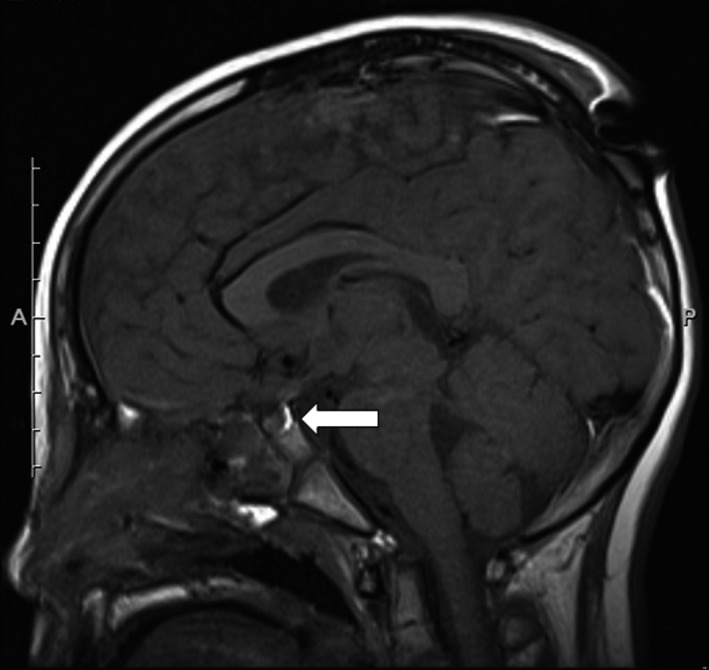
Head magnetic resonance imaging of a 9‐year‐old boy who was injured in a car accident. The T1‐weighted image shows the normal appearance of a high intensity signal in the posterior pituitary lobe (arrow).
